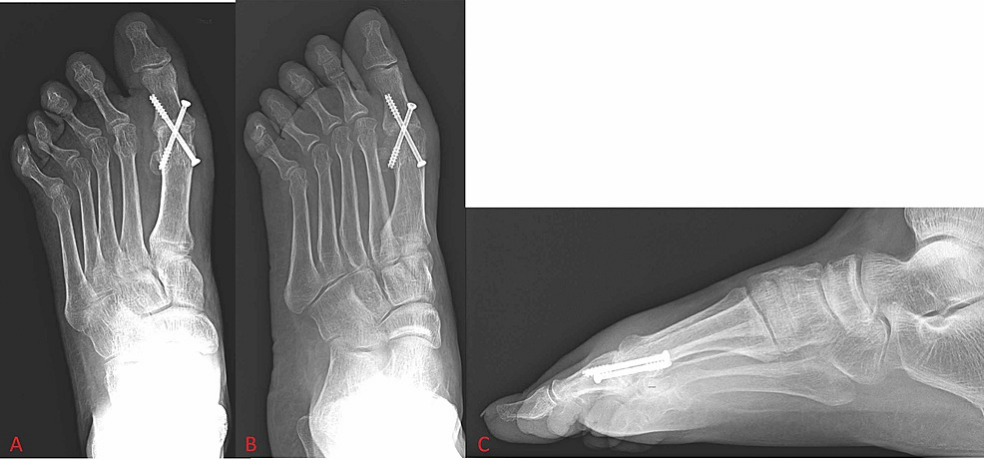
Foot X-ray shows crossed-screw fixation of the first metatarsophalangeal joint. Figure 1A and Figure 1B show anterior-posterior and oblique views of the foot, respectively. Figure 1C shows a lateral view of the foot.
